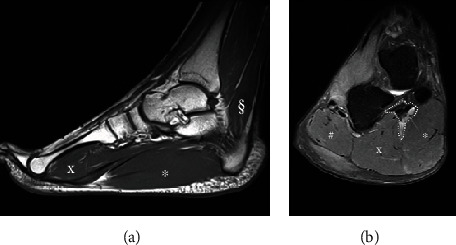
Sagittal (a) and coronal (b) MRI scans show hypertrophy of abductor hallucis (∗), flexor digitorum brevis (x), abductor digiti minimi (#), and accessorius soleus muscle (§).
